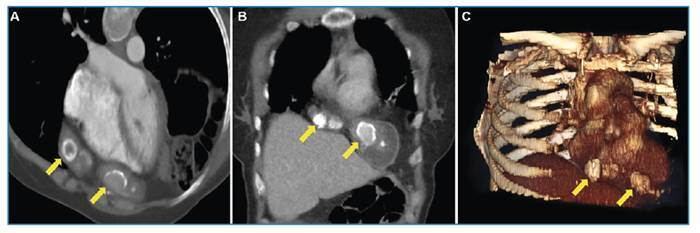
Contrast-enhanced thorax CT images obtained in the axial oblique (A) and coronal (B) planes, as well as 3D oblique anterior-posterior reconstruction images (C), showing a central hyperdense lesion (arrows) in the pericardial area extending to the diaphragm.
